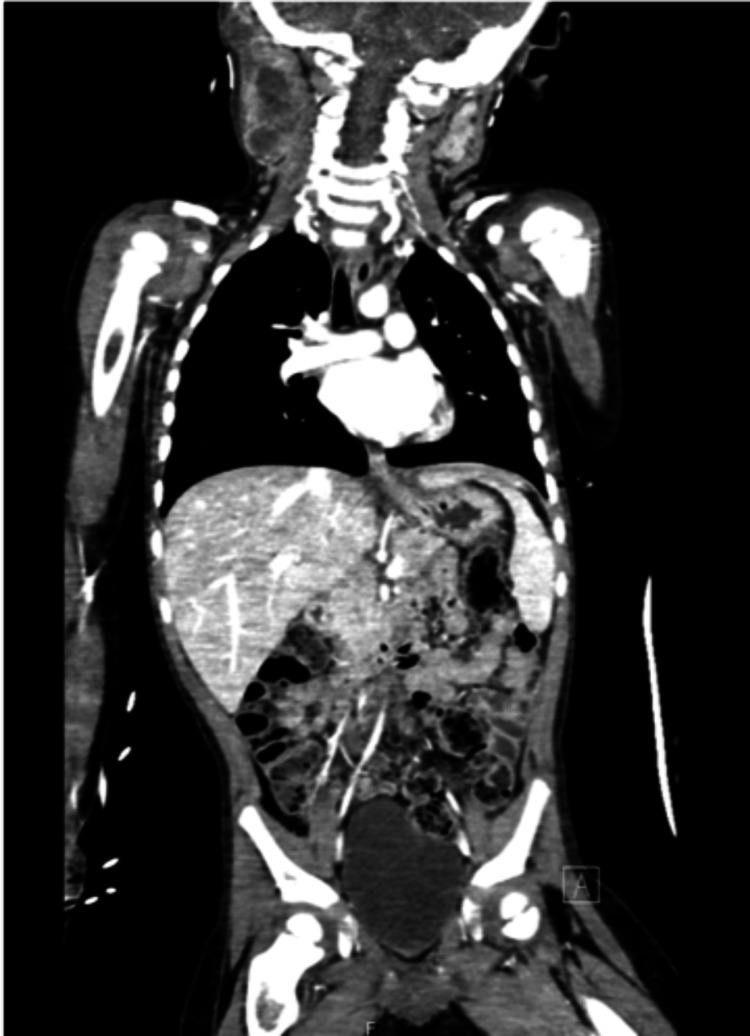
Head, cervical, thoracic, abdominal and pelvic CT images showing a single large cervical mass.
